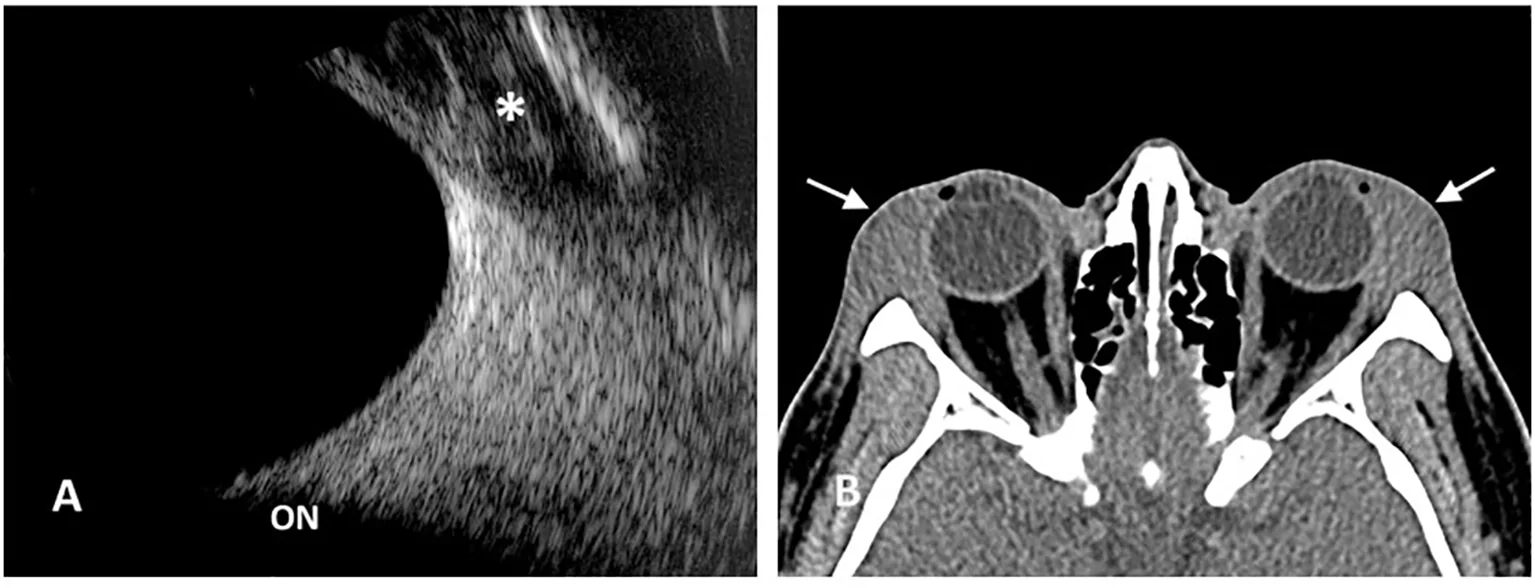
Sarcoid Dacryoadenitis. (A) Longitudinal B-scan right eye aimed superotemporally shows a well-defined, homogeneous, medium reflective enlargement of the gland (asterisk). (B) Axial CT scan shows bilateral lacrimal gland enlargement (arrows). *ON*, optic nerve. The asterisk (*) is used to label an area of the figure.
